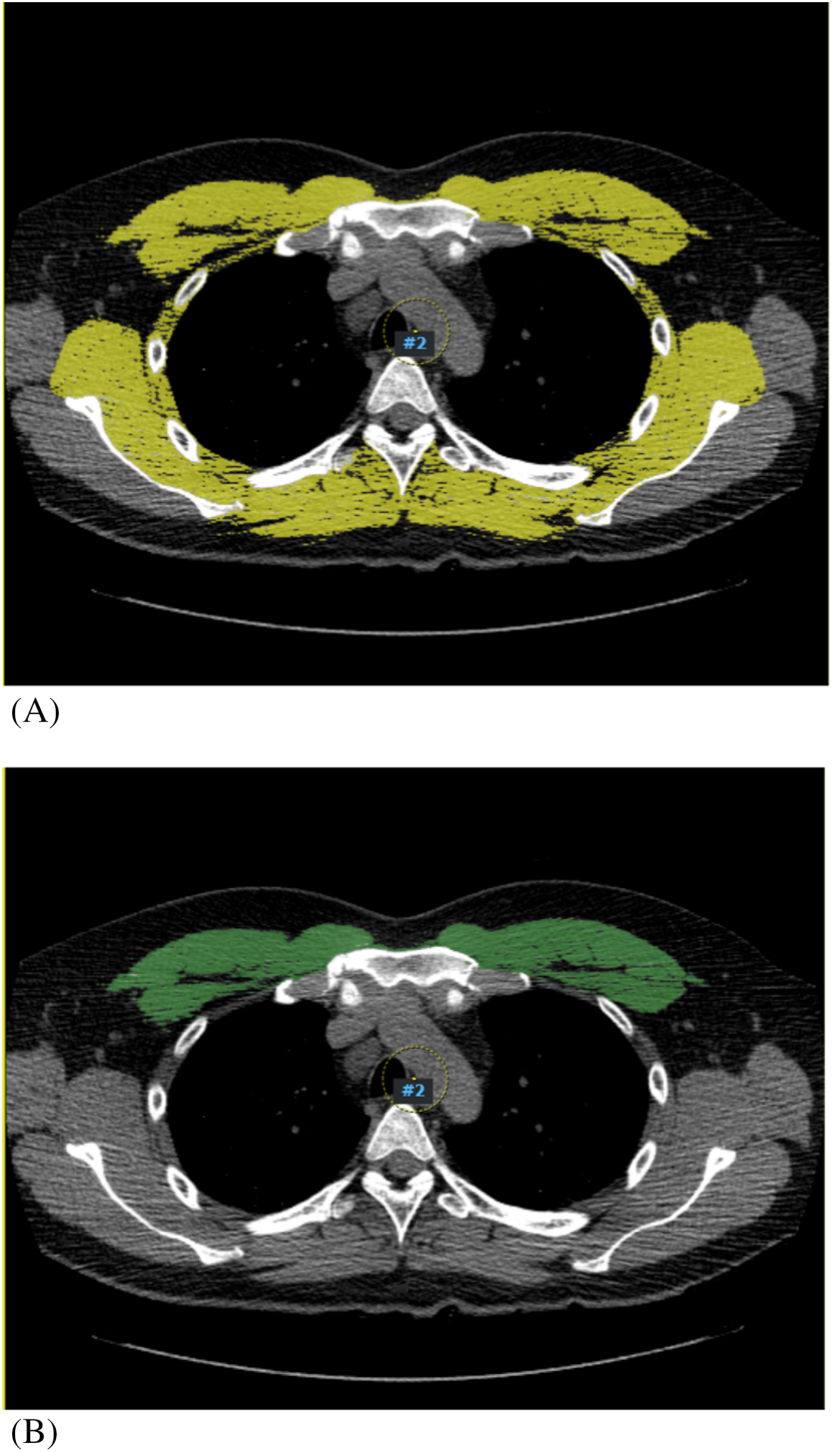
Sample axial CT images of the fourth thoracic vertebral region. (*A*) Pectoralis, intercostalis, paraspinal, serratus, and latissimus muscles (T4_CSA_) are in yellow. (*B*) Pectoralis muscles (PM_CSA_) are in green.
